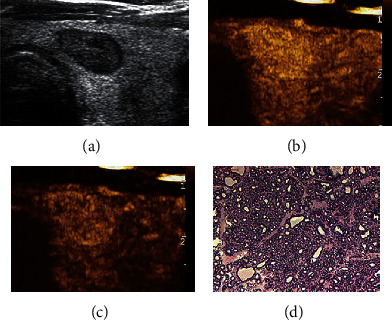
Adjustment of C-TIRADS classification of thyroid nodule with hyperenhancement in CEUS. A 61-year-old male patient with a left thyroid nodule, maximum diameter of 16 mm, which was originally classified as C-TIRADS 4A before the adjustment based on the CEUS results. The nodule was reclassified as C-TIRADS 3 after the adjustment. (a) Two-dimensional gray scale. (b) Hyperenhancement at 17 s in CEUS. (c) Still hyperenhancement at 37 s in CEUS. (d) Diagnosed as thyroid follicular adenoma (HE × 100). CEUS: contrast-enhanced ultrasound; C-TIRADS: the Chinese-Thyroid Imaging Report and Data System.
